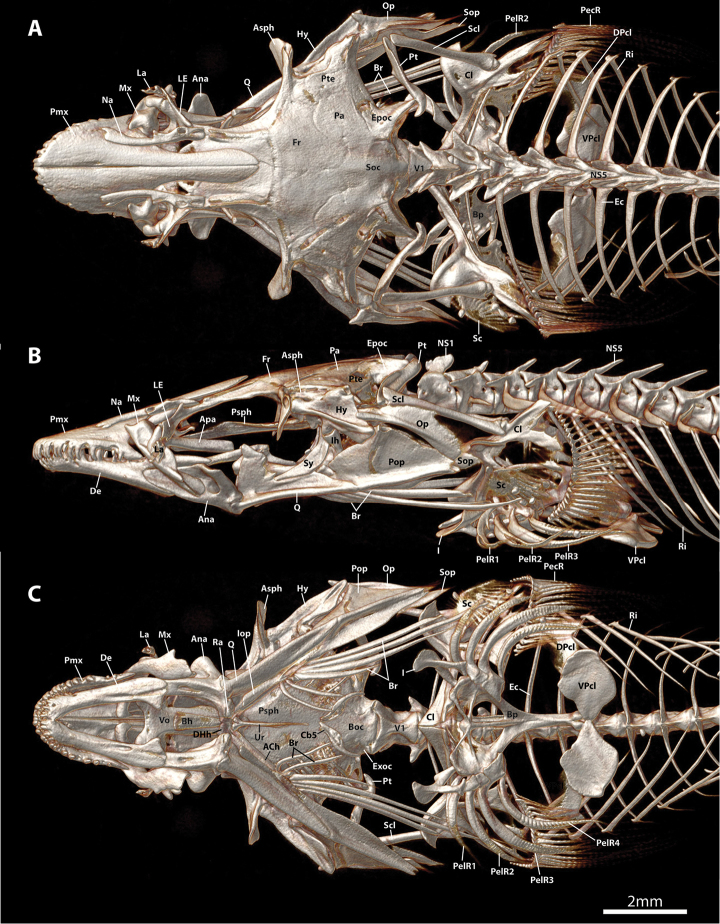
CT scanned anterior skeleton, including cranium, paired-fin girdles and abdominal region of vertebral column, of *Dellichthys
